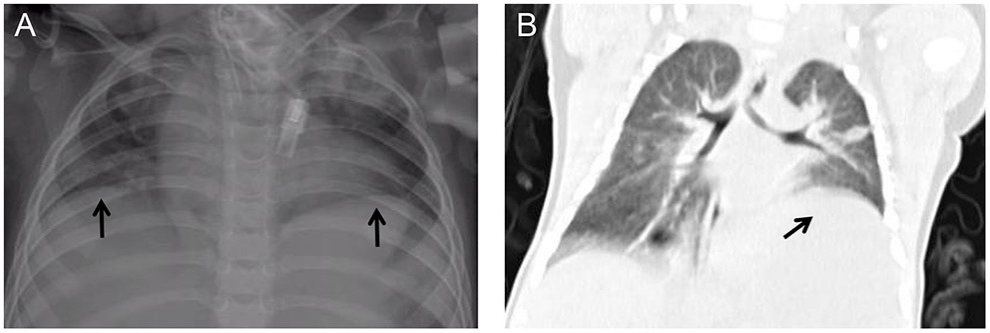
Post-operative chest X-ray (A) and computed tomography in coronal view (B) showing the unilateral diaphragmatic elevation (arrows).
